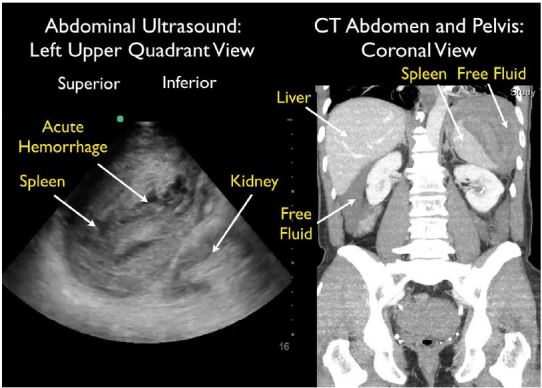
Splenic rupture after colonoscopy. Left image, ultrasound. Right image, computed tomography scan (CT).
